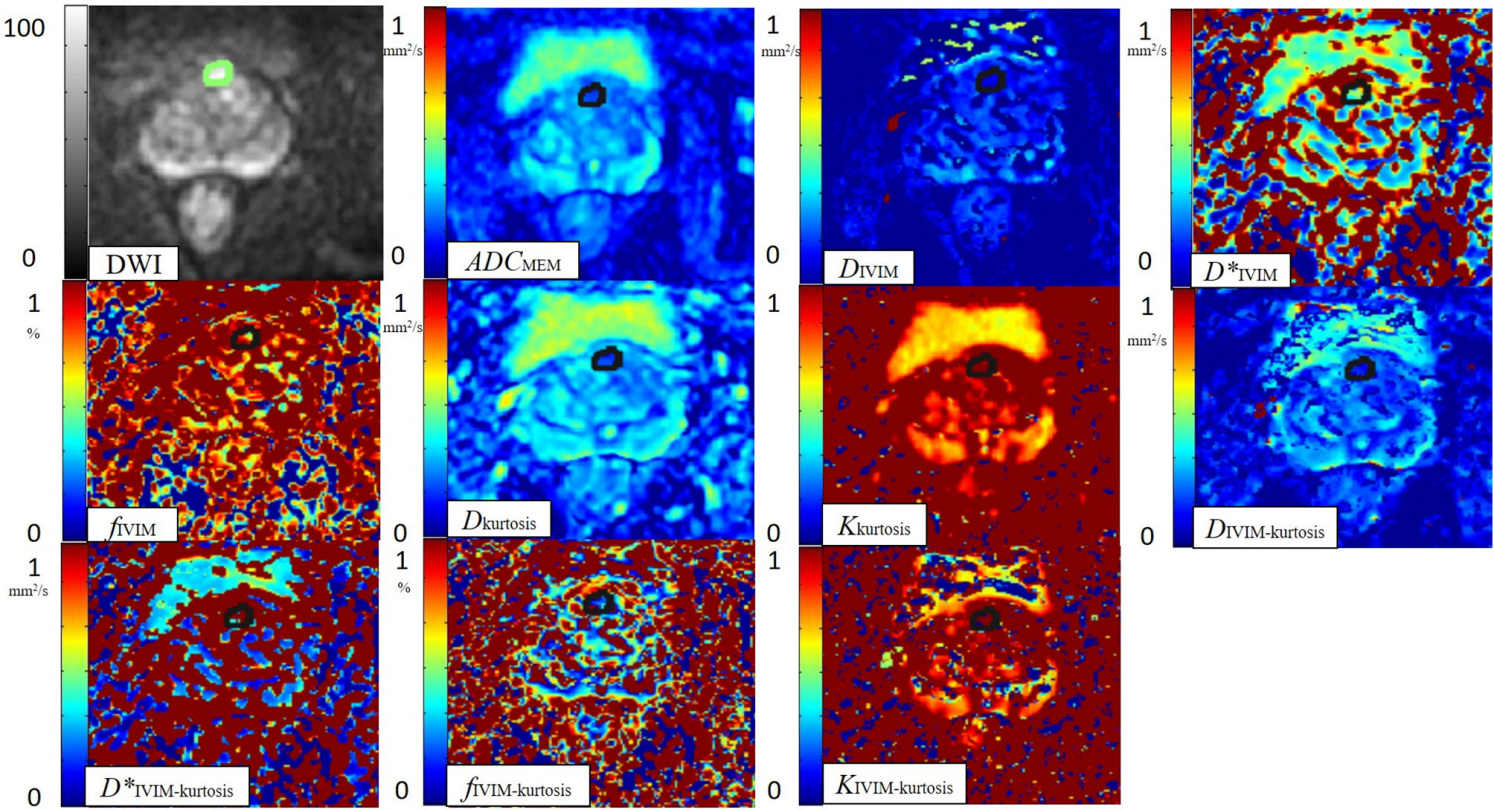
One case with BPH. A 60-year-old man with BPH. ROI was set in BPH on the DWI image. ADC_MEM_, *D*_IVIM_, *D_IVIM_, *f*_IVIM_, *D*_kurtosis_, *K*_kurtosis_, *D*_IVIM−kurtosis_, *D_IVIM−kurtosis_, *f*_IVIM−kurtosis_, and *K*_IVIM−kurtosis_ value maps. The BPH tissue showed different signals notably from normal tissues on the DWI value map. The BPH tissue showed a slightly low signal on the ADC_MEM_ (0.87 × 10^−3^ mm^2^/s), *D*_VIM_ (0.45 × 10^−3^ mm^2^/s), *D*_kurtosis_ (1.34 × 10^−3^ mm^2^/s), and *D*_IVIM−kurtosis_ (1.22 × 10^−3^ mm^2^/s) value maps. The BPH tissue seemed to be similar to normal tissues on the *D_IVIM_ (4.09 × 10^−3^ mm^2^/s), *f*_IVIM_ (46.17%), *K*_kurtosis_ (0.96), *D_IVIM−kurtosis_ (1.65 mm^2^/s), *f*_IVIM−kurtosis_ (15.25%), and *K*_IVIM−kurtosis_ (1.01) value maps.
